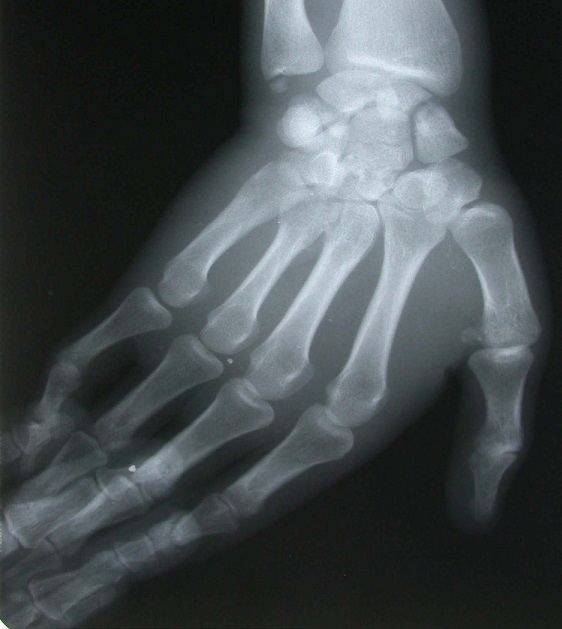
Radiographies du poignet (face-profil): fracture du scaphoïde; du capitatum; du pyramidal et de l’apophyse styloïde cubitale, sans luxation des os du carpe
